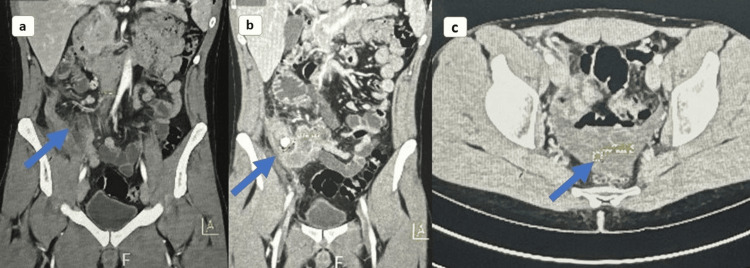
CT abdomen and pelvis, coronal (a, b) and axial section (c), showing hyper-enhancing terminal ileum of the right lower quadrant, appendicolith, and enlarged appendix.
